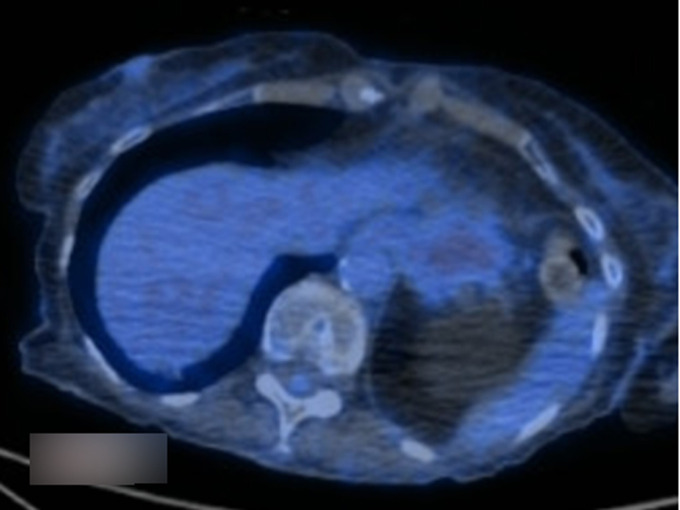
positron emission tomography (PET) scan and a computed tomography (CT) scan showing vasculitis of aorta
